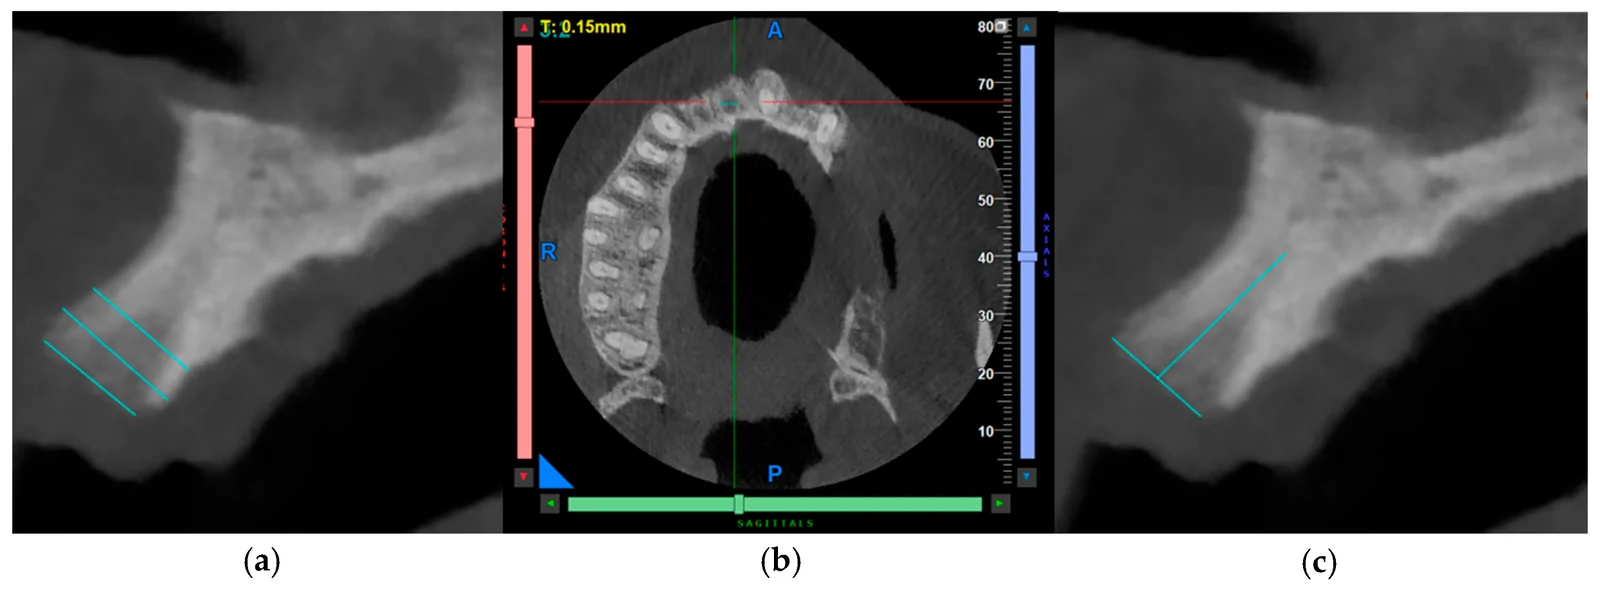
(a) Measurements taken of the healed socket using CBCT at the crest, 1 mm from the crest, and 3 mm from the crest; (b) mesiodistal dimension; (c) socket depth.
